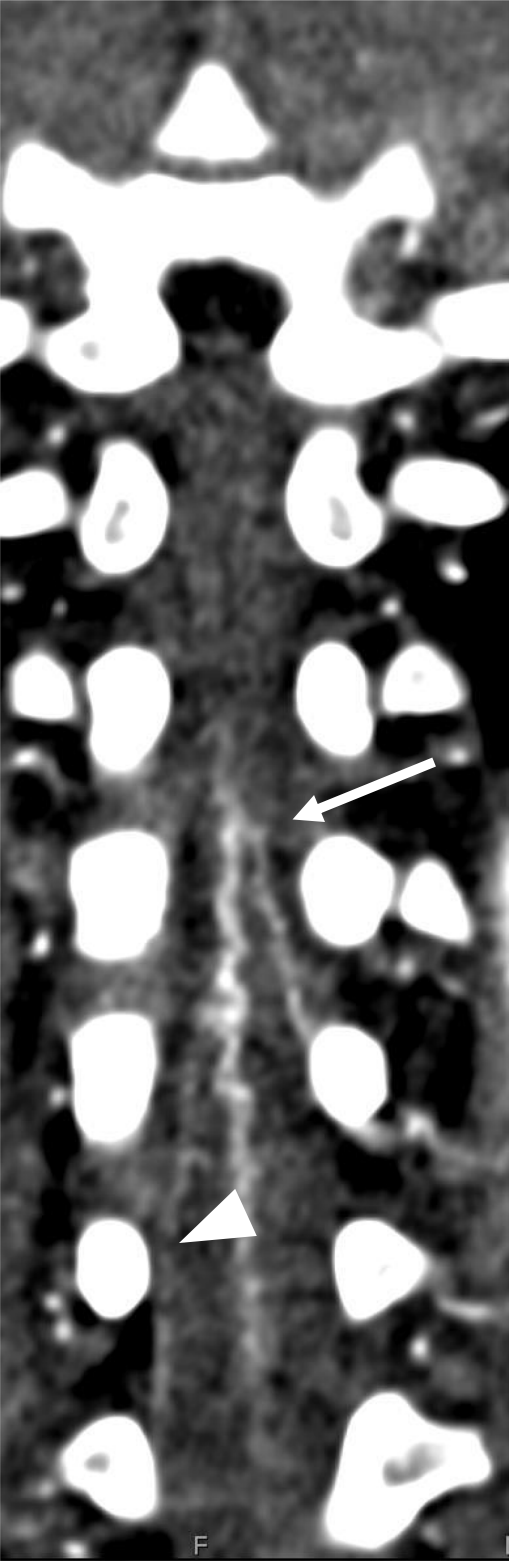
A 23-month-old male with more than one artery feeding the anterior spinal artery. Coronal CTA chest in soft tissue window. The dominant artery on the left (*arrow*) arising from L1 was determined as the artery of Adamkiewicz. The smaller artery on the right (*arrowhead*) was identified as a lesser radicular artery
